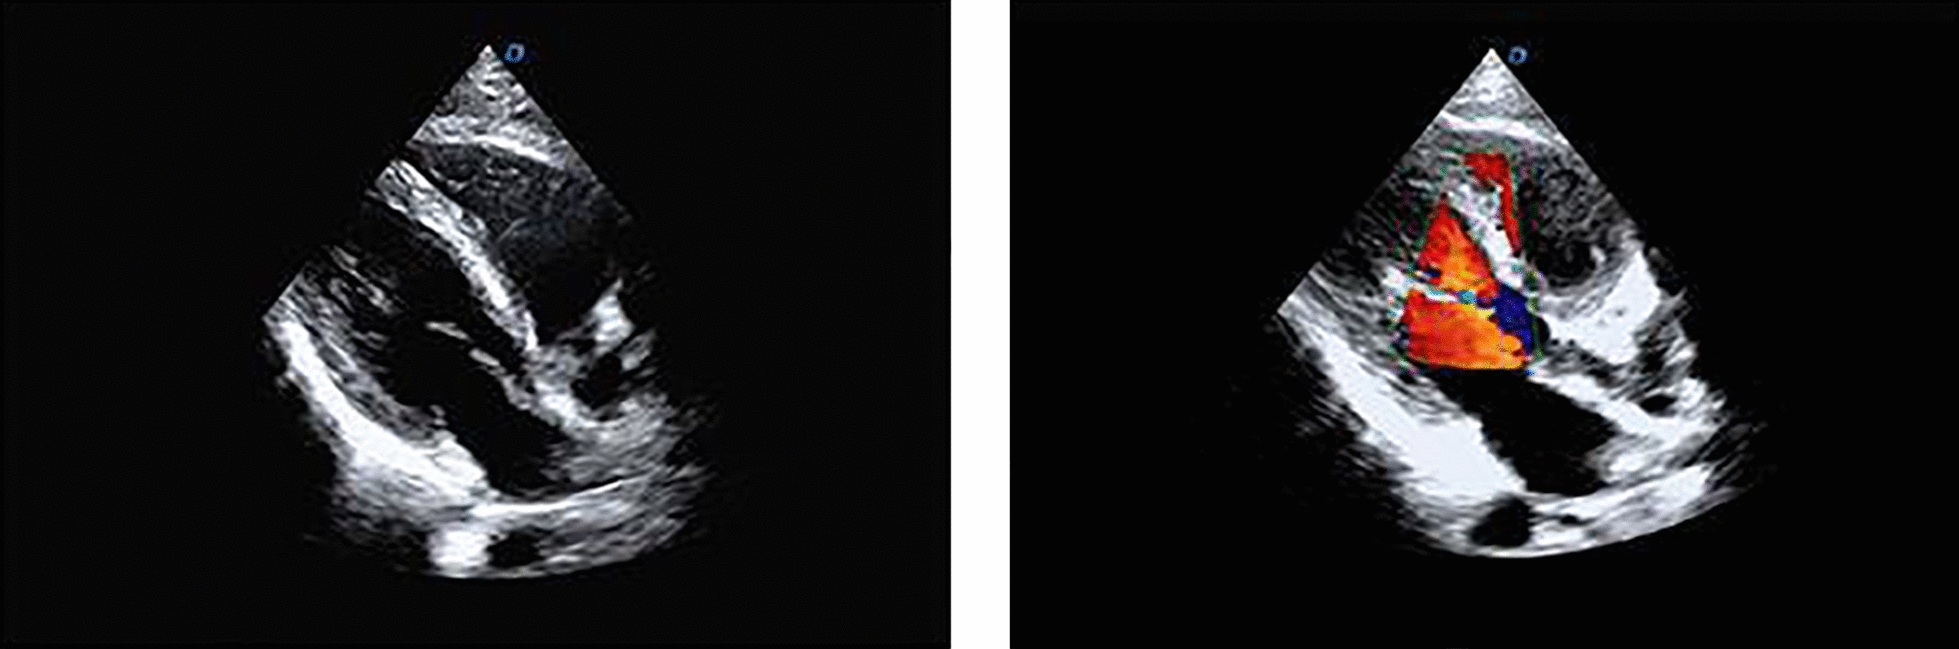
Ultrasound flow imaging of the heart
